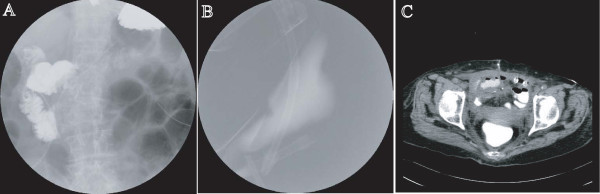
Abdominal imaging eight days after the third operation. (A) Both poor flow of contrast medium and intestinal distention are evident. (B) Radiograph of the drain bag demonstrating the presence of the contrast medium taken orally eight days after the third operation. (C) The day after this examination, fluid collection with extravasation of contrast medium is visible.
